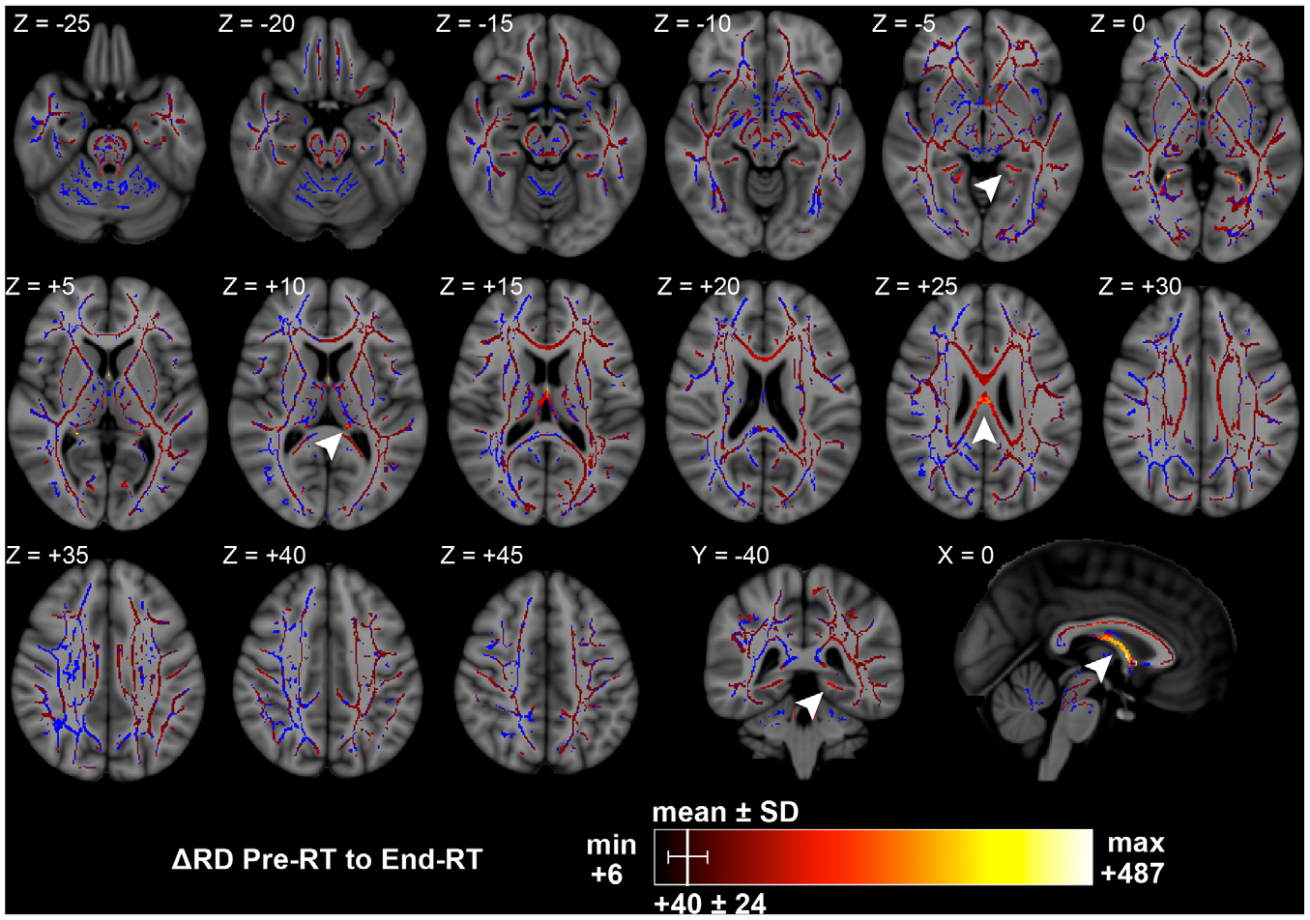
Significant changes in RD from pre-RT to end-RT. Arrowheads: Z = −5, left inferior cingulum; Z = +10, left fornix crus; Z = +25, corpus callosum body; Y = −40, left inferior cingulum; X = 0, fornix columns. Significant results per color chart, blue is TBSS skeleton without significant results. Depicted on MNI ICBM152 standard brain T1-weighted image [34]. Units are µm^2^/s.
